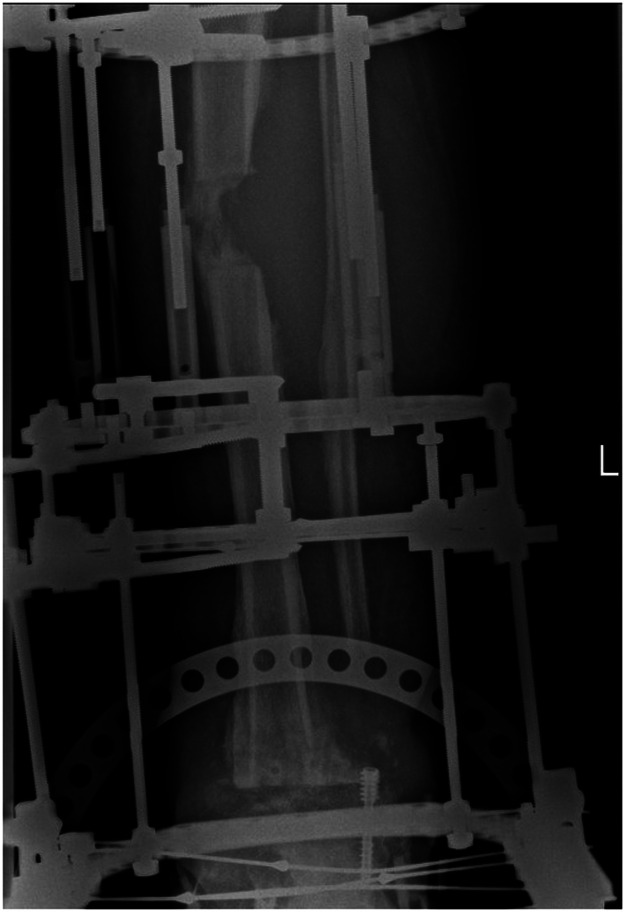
Radiographic image showing the deformity in the bone regenerate caused by the pressure exerted by the pseudoaneurysm.
